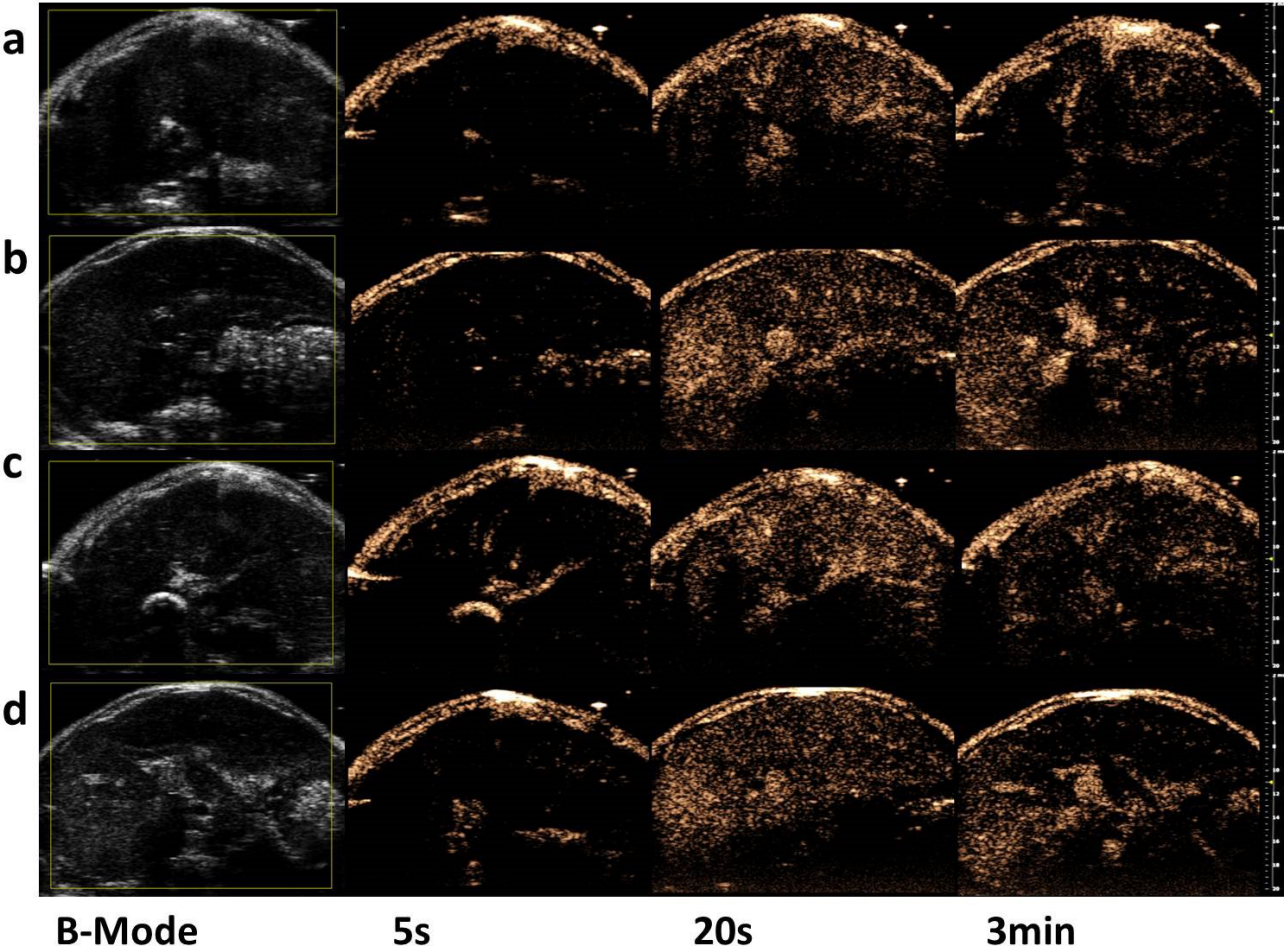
In vivo ultrasound image enhancement. Ultrasound-enhanced images of liver before and after vein injection of different SNBs and control MBs at 5 s, 20 s and 3 min. (a) SNB 1, (b) SNB 2, (c) SNB 3 and (d) control MBs.
